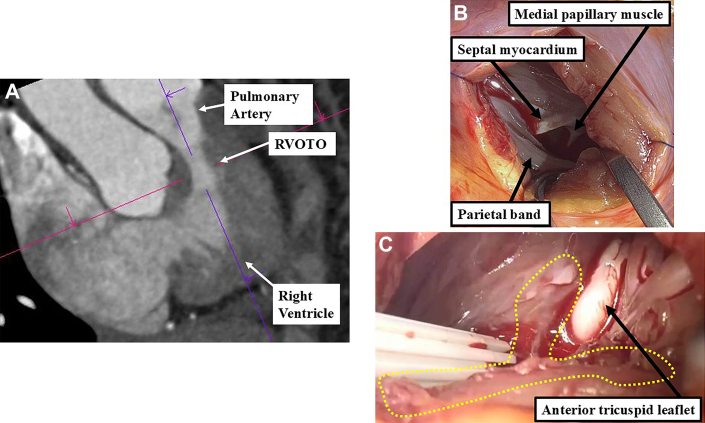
Findings Related to the RVOTO (A) Preoperative CT suggested a stenotic lesion below the pulmonary valve indicative of RVOTO. (B) The main causes of RVOTO were identified as the parietal band and hypertrophied interventricular septum. (C) Resection of the parietal band and anterior right ventricular wall relieved the RVOTO. CT = computed tomography; RVOTO = right ventricular outflow tract obstruction.
